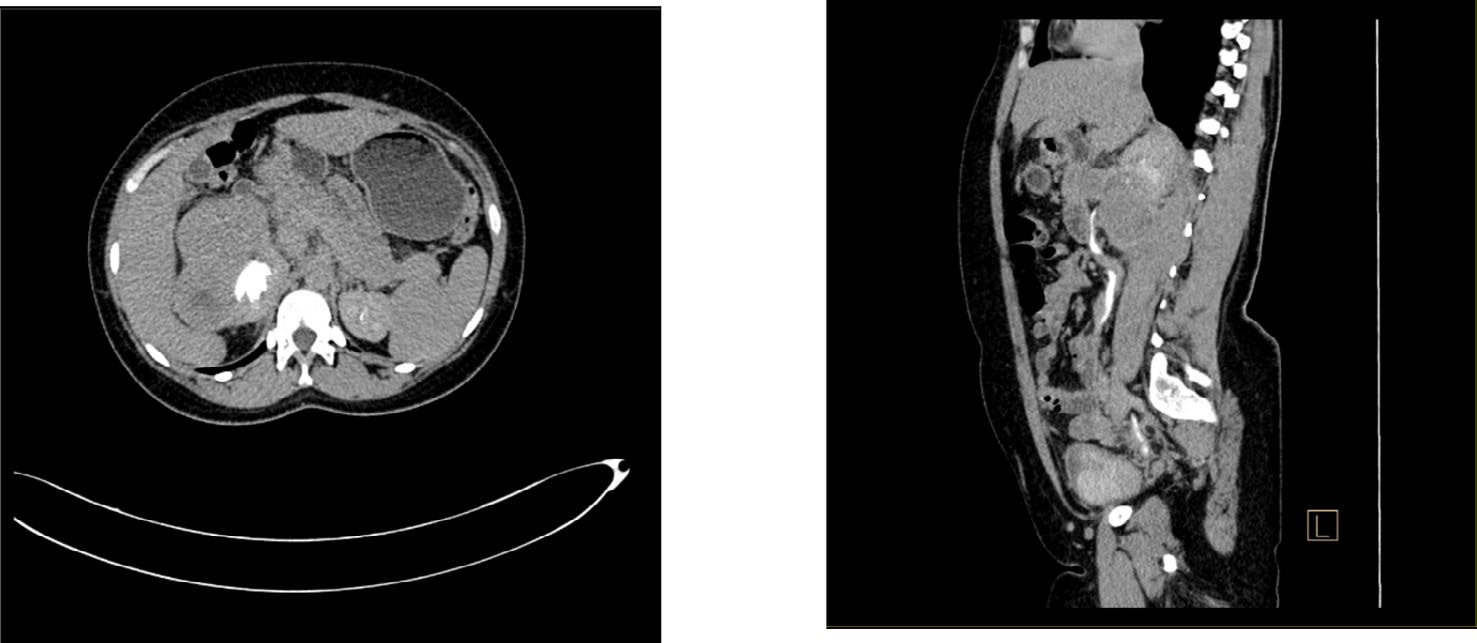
Contrasted axial and coronal delayed CT images of the abdomen showing normal excretion.
